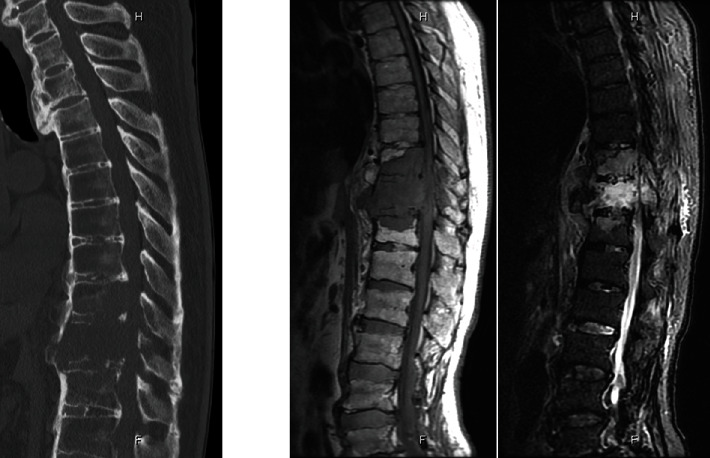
He presented with acute onset of severe back pain. CT and MRI reveal a fracture of the metastatic lesion in T10–T12.
